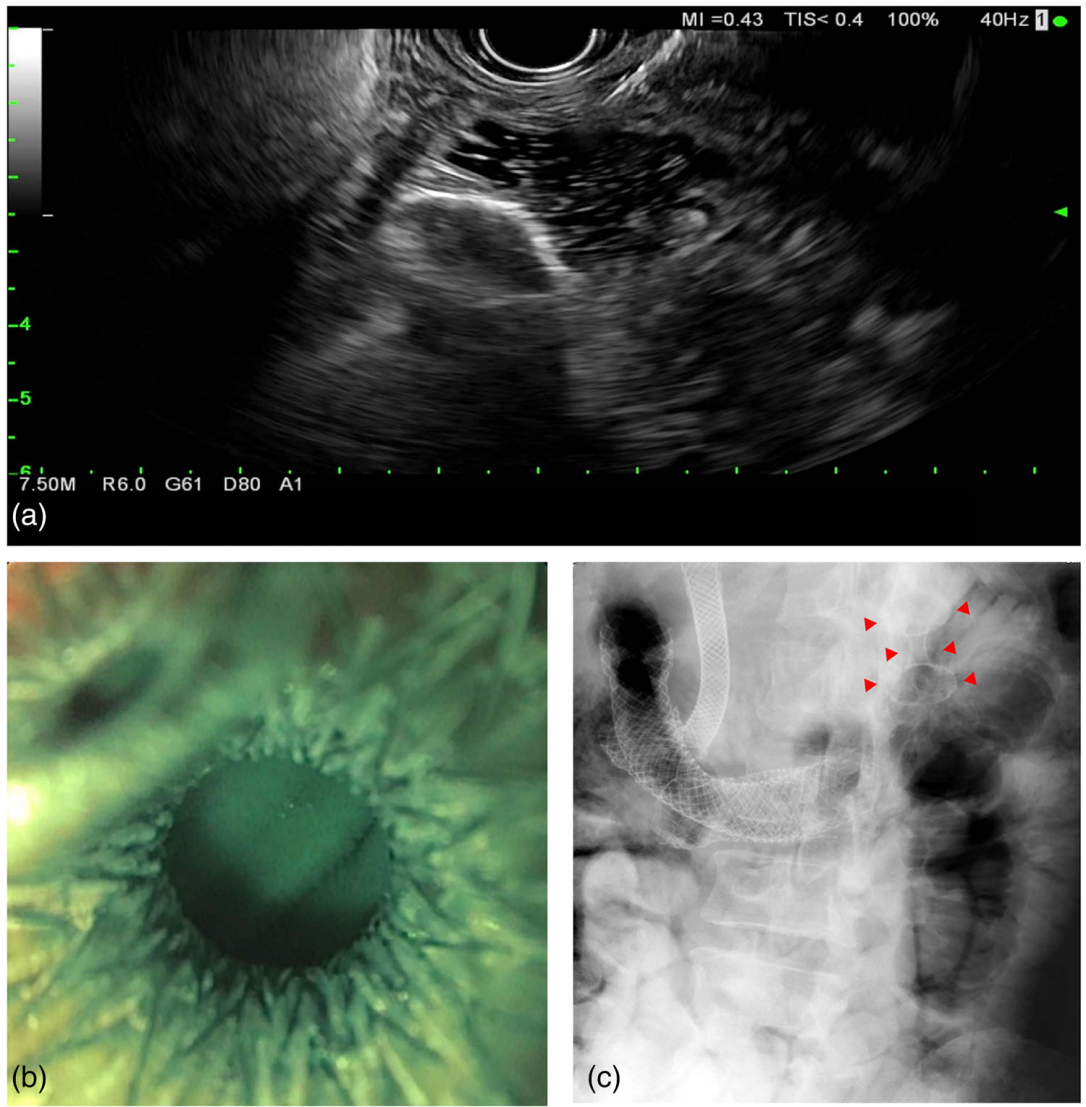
Endoscopic ultrasound‐guided gastrojejunostomy procedure using nasojejunal tube‐assisted technique and an electrocautery‐enhanced lumen apposing metal stent. Stent placement under real‐time endosonographic imaging (a). Endoscopic visualization of the small bowel after successful stent placement with drainage of blue‐tinted sterile saline (b). Radiographic image confirming positioning of the lumen apposing stent distal to the enteric stents ‐ indicated by red arrows (c).
